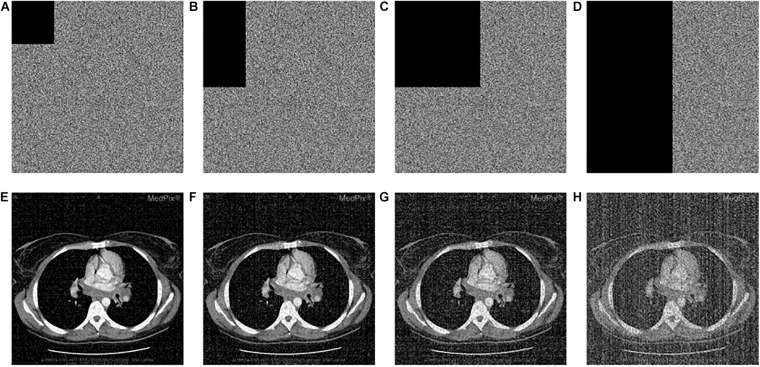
Occlusion attack: the data loss of encrypted “CT-chest” with (A) 1/16, (B) 1/8, (C) 1/4, and (D) 1/2; corresponding decrypted image (E–H) in accordance with (A–D).
